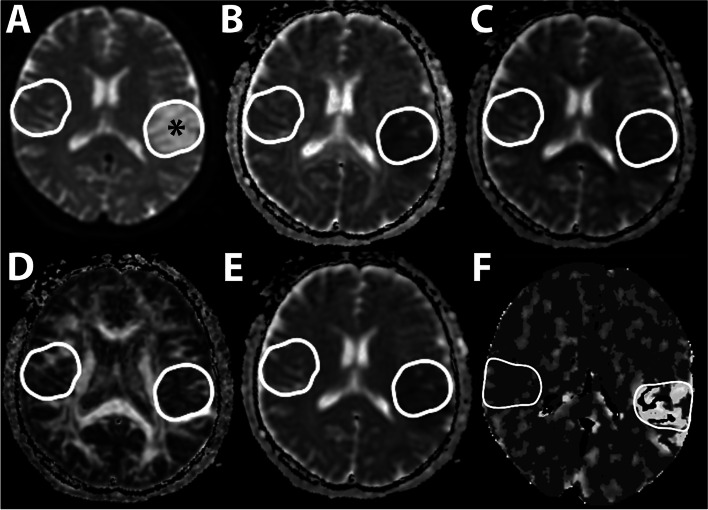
Advanced brain imaging. The most representative slice was selected from a set of diffusion-tensor imaging and CT perfusion scans. The region of interest is outlined on the affected side (*) and is mirrored to the contralateral hemisphere. A. Raw DTI scan, b-value = 0 s/mm^2^. B. Axial diffusivity map. C. Radial diffusivity map. D. Fractional anisotropy map. E. Mean diffusivity map. F. Permeability–surface area product map
